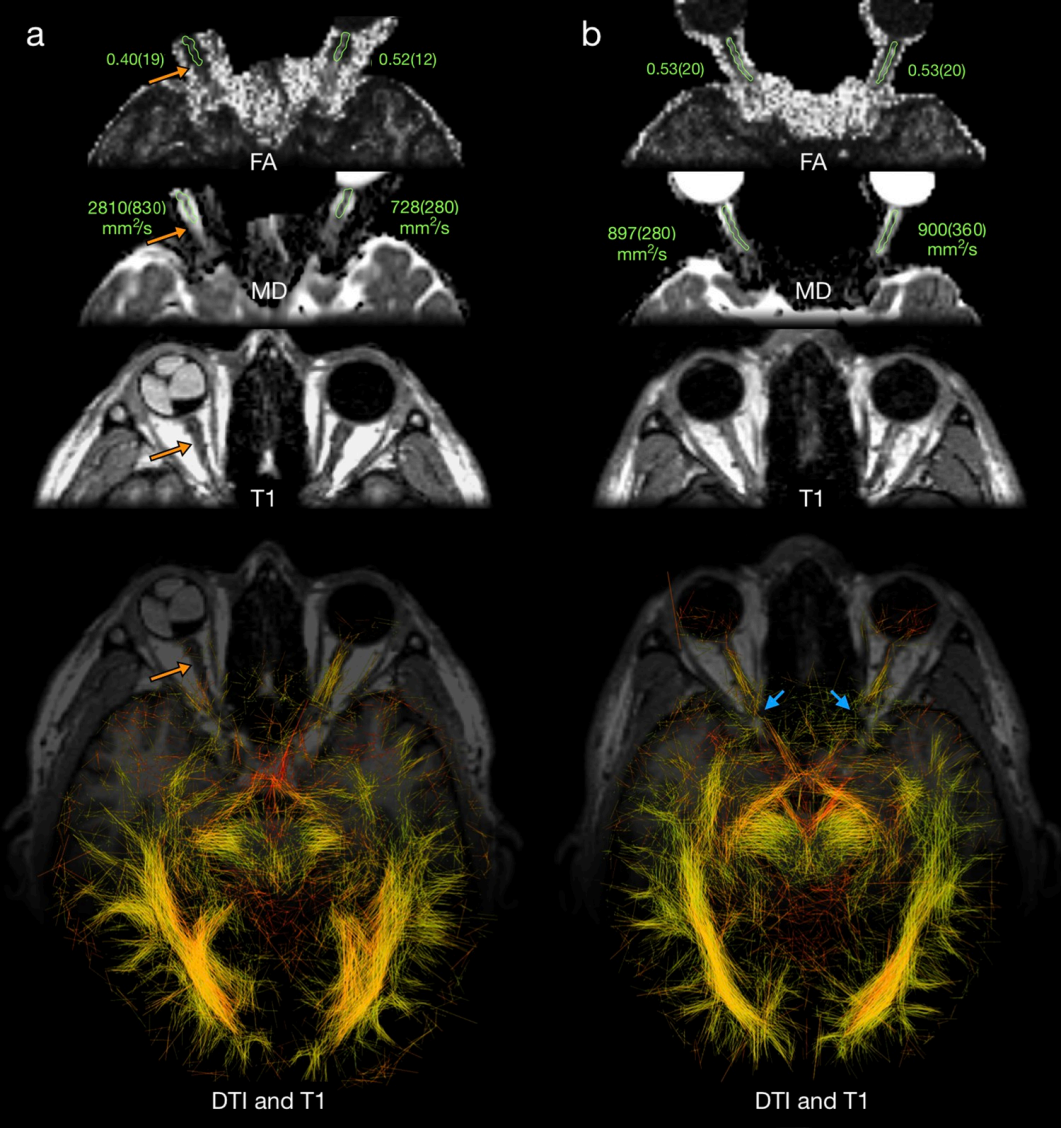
Visual pathway of male subject with right optic nerve atrophy (orange arrows) (a) and for healthy male subject (b) visualized with the new DTI algorithm superimposed on the same T1 image. Optic nerves are also presented on diffusion scalar maps FA and MD, and on morphological T1-weighted images. Actual values of FA and MD measured in a region of interest containing the optic nerve are presented in green. Blue arrows: optic canal and locations of visible discontinuities. Orientation convention: radiological. Values of *α*, *β*, *γ* and *σ* used in visualization were 250, 1000, 1.2 and 0.2, respectively.
